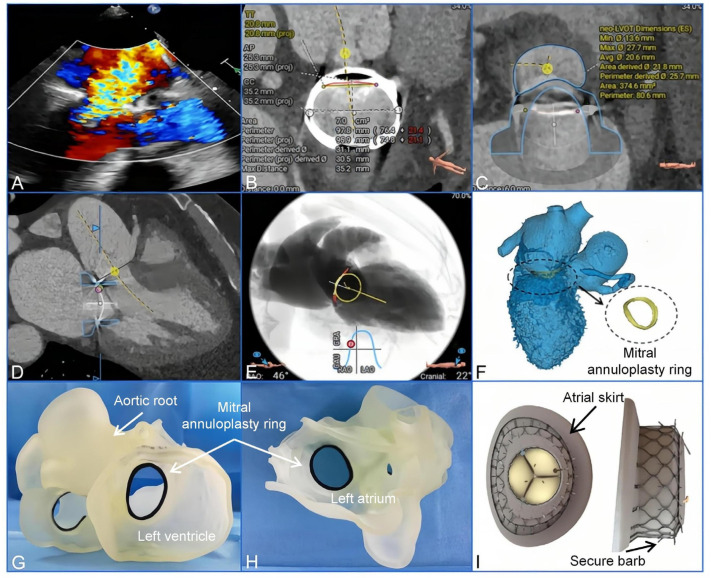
Preoperative imaging assessments and 3-dimensional-printed model reconstructions were used to formulate the procedural plan. (A) Preoperative echocardiography revealed severe mitral regurgitation. (B–E) Preoperative computed tomography angiography was used to access the mitral valve annulus and the left ventricular outflow tract. (B) The area of the mitral annuloplasty ring was 7.0 cm^2^. (C,D) The area of the neo-left ventricular outflow tract after implanting the bioprosthesis of the Mi-thos system was 374.6 cm^2^, and no obstruction occurred. (E) The projection angle of the released implanted valve was RAO46, CAU22. (F) Three-dimensional reconstruction and calculation of landing-zone structures were based on computed tomography angiography data. (G,H) The preoperative 3-dimensional printed model in the left atrial and the ventricular views. (I) With a D-shaped design, the bioprosthesis of the Mi-thos system fit the saddle-shaped mitral annulus.
